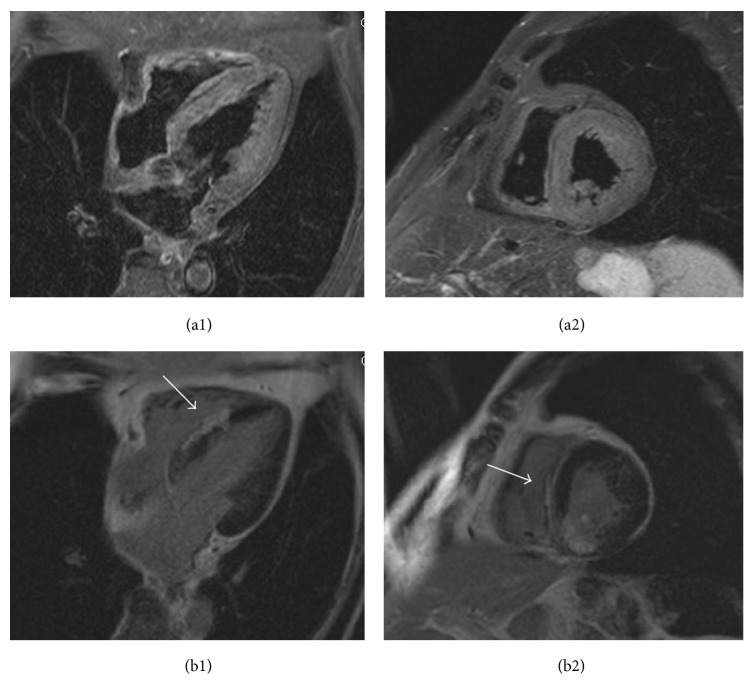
CMR at 4 months later demonstrated resolution of the hematoma with epicardial LGE. (a) T2-weighted image. (b) LGE image.
